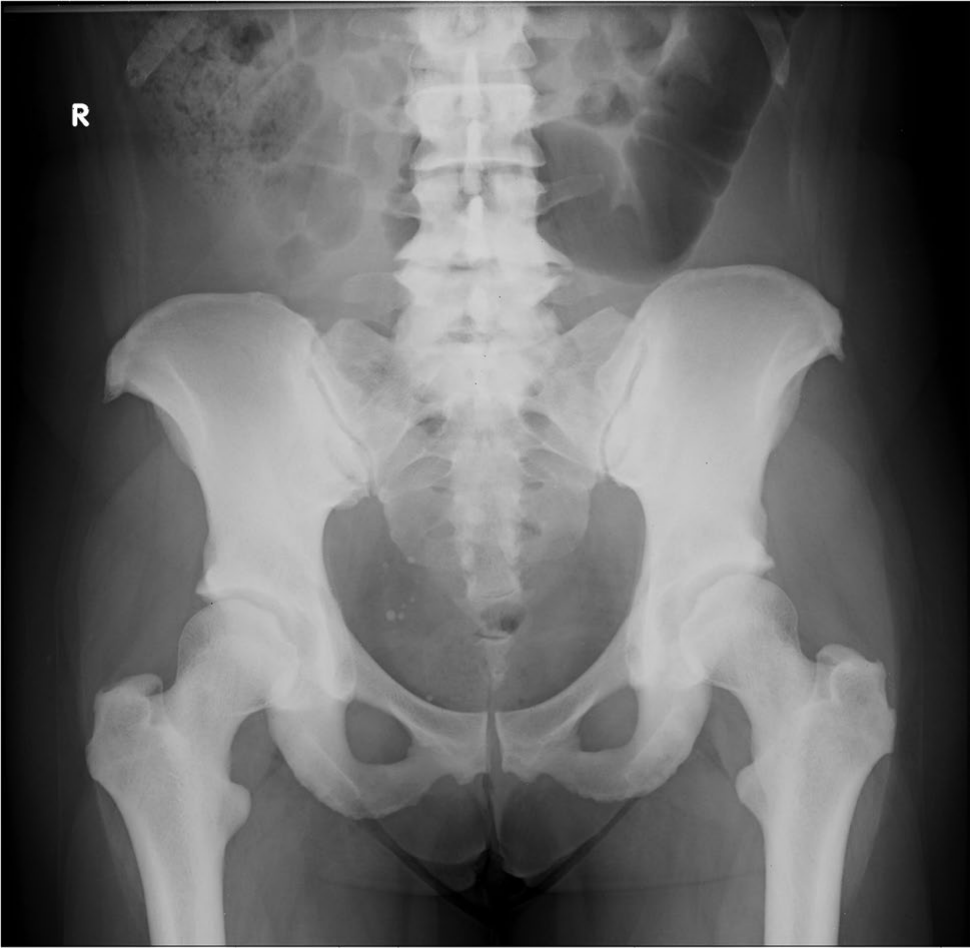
X-rays showing endosteal hyperostosis of femurs and osteosclerosis of hip and vertebrae. Written informed consent was obtained from the patient for publication of the radiograph
